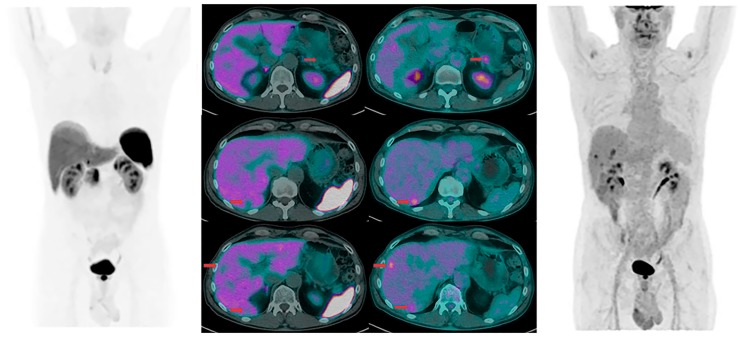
Sixty-seven-year-old male patient with newly diagnosed pancreatic neuroendocrine tumors (NET) with liver metastases. All lesions are positive at [^18^F]FDG PET/CT (right) and negative at [^68^Ga]Ga-DOTA-NOC PET/CT (left), indicating a more aggressive and un-differentiated disease.
